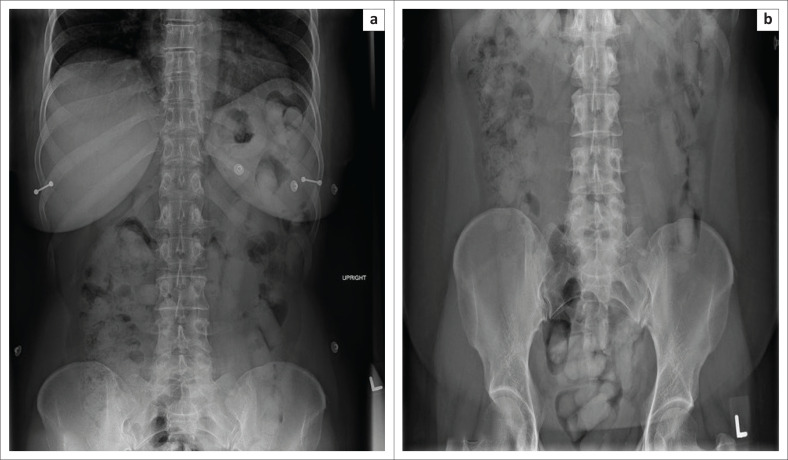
(a and b) Abdominal radiographs of packages containing narcotic materials in a 45-year-old woman.
